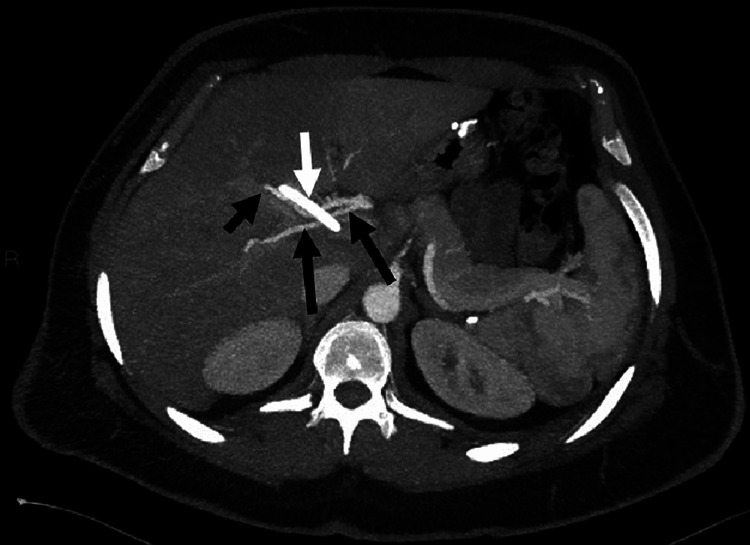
Axial contrast-enhanced CT arterial phase MIP image shows crossing right hepatic artery branches (black arrows) against the stented common hepatic duct (white arrow). Regression of intrahepatic biliary dilatation post stenting.
